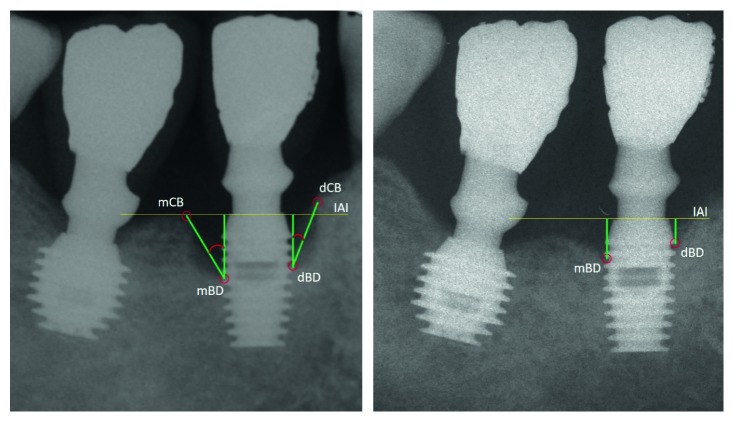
Radiographs were digitalized and used for measurements of bone-level changes between the baseline and last follow-up. mBD: mesial bone defect, dBD: distal bone defect, mCB: mesial crestal bone, dCB: distal crestal bone, IAI: implant-abutment interface.
